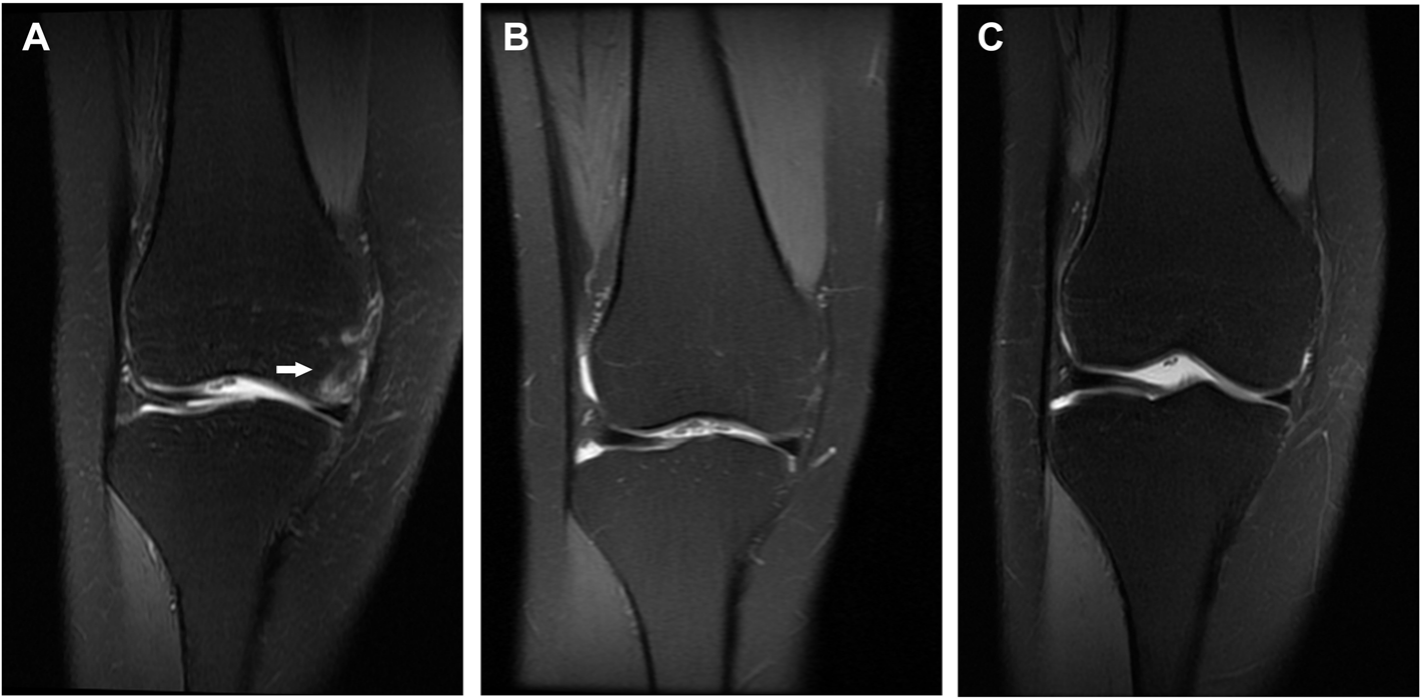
A patient with BMLs (arrow) on MRI: hyperintensity on fat-suppressed T2-weighted image (a). After calcitonin treatment, no abnormal signal intensity was found at 4-week follow-up (b) and 3-month follow-up (c). *BMLs* bone marrow lesions, *MRI* magnetic resonance imaging
